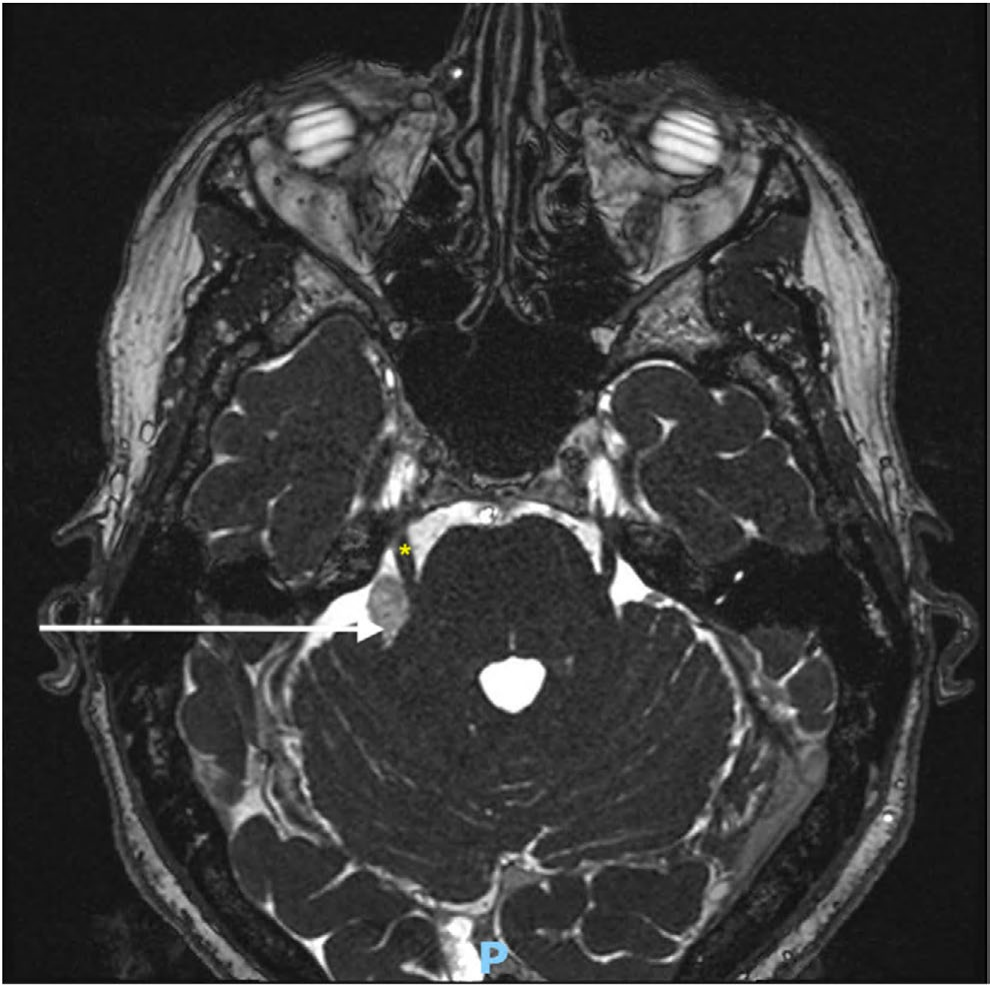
Preoperative MRI (T2‐weighted sequence) showing the vestibular swhannoma (white arrow) and its proximity with trigeminal nerve (yellow asterisk).
